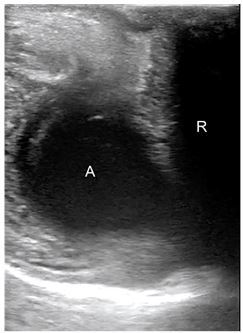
Sagittal sonographic view of the upper anal canal with space-occupying hypoechoic lesion (A) adjacent to the posterior canal and possible connection to the anal canal (R). rectal ampulla; *A*, abscess.
